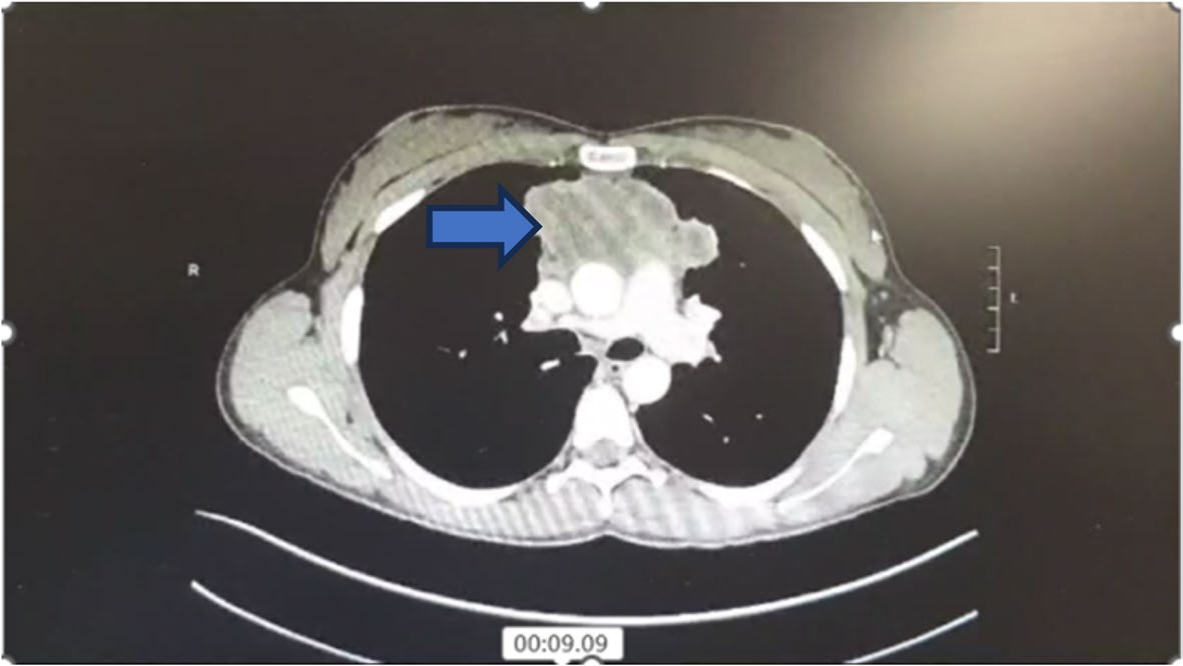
CT-Scan showing mediastinal mass (blue arrow)
